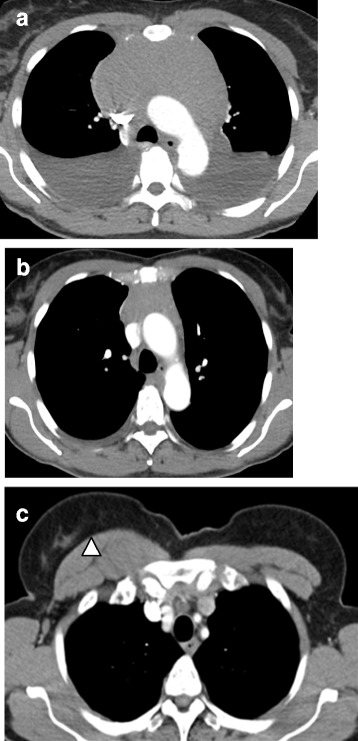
76-year-old woman with chronic lymphocytic leukemia with 11q deletion and unmutated IGHV. a Axial CT images of the chest acquired during arterial phase at the time of initial presentation shows a large anterior mediastinal mass measuring up to 10 cm in its largest dimension with bilateral moderate pleural effusions. b Axial CT image of the chest obtained during arterial phase 4 months after initiating R-CHOP demonstrates significant decrease in size of the anterior mediastinal mass and improvement of bilateral pleural effusion. c Axial CT image of the chest obtained during arterial phase 4 years later demonstrates a new mass in the right sub pectoral region measuring up to 3.5 cm in its largest dimension suspicious for CLL (arrowheads). The lesion was biopsied and was consistent for recurrence of CLL
